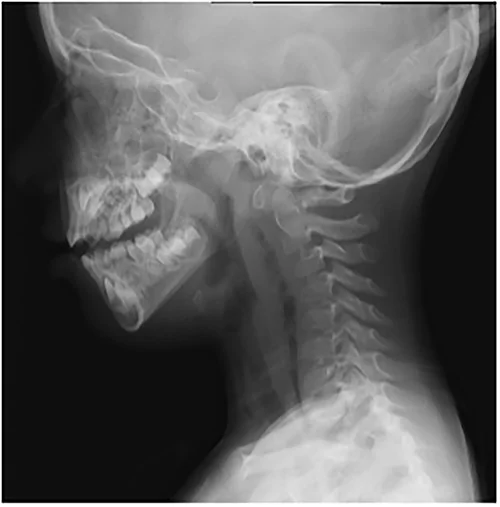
Postoperative lateral soft tissue neck X-ray showing thickened retropharyngeal area with air foci.
